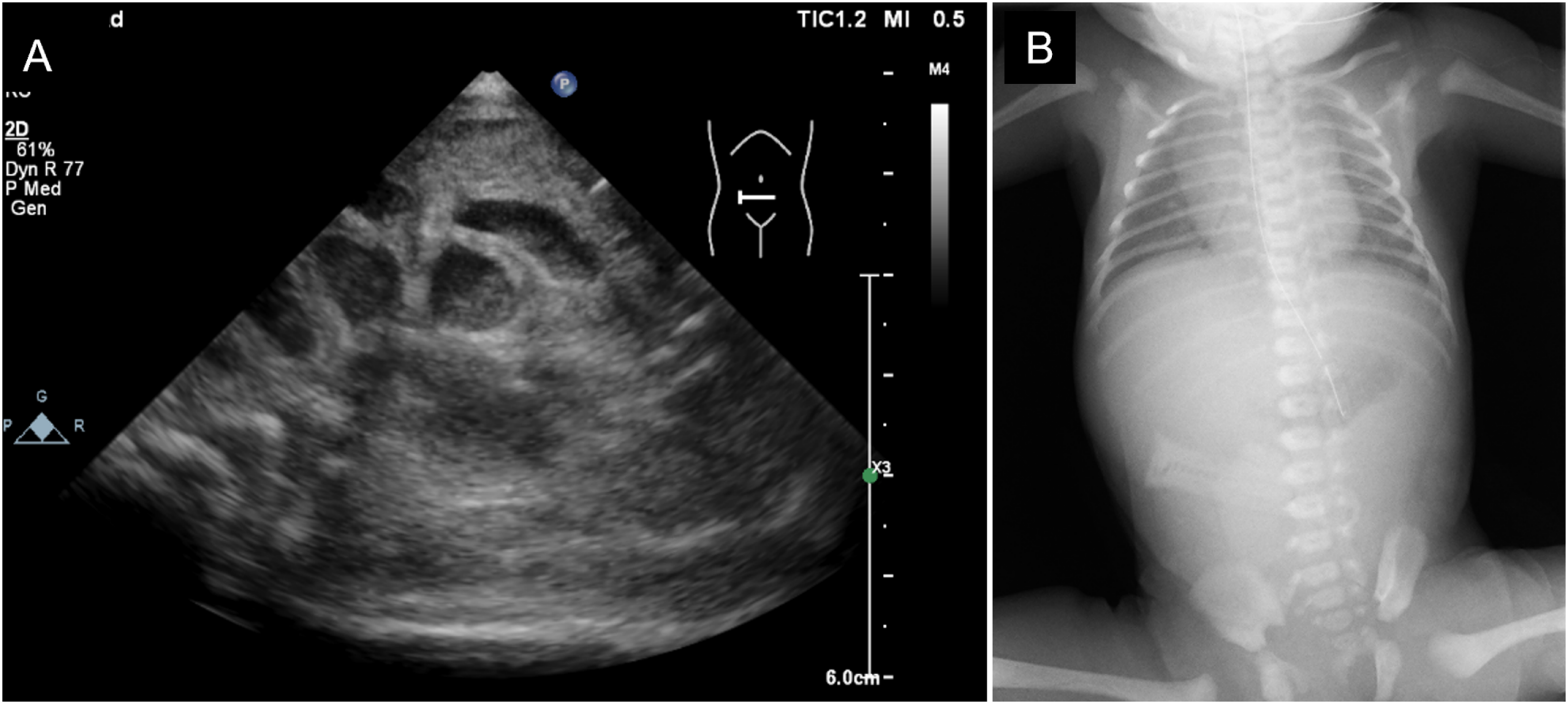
Imaging examination on admission. (A) Abdominal ultrasonography. (B) x-ray of the chest and abdomen.
